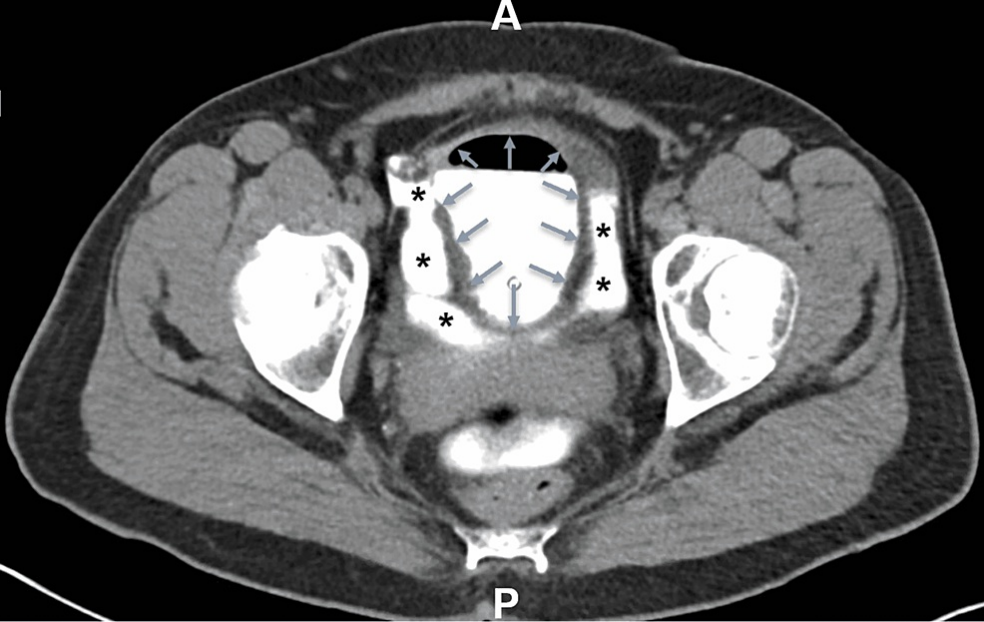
CT cystogram demonstrating contrast extravasation into the intra-peritoneal space. The grey arrows mark the boundaries of the bladder wall. The black asterisks signify contrast extravasation. Post-surgical biopsy sample of the anterolateral bladder wall revealed focal denudation of the urothelial mucosa.
